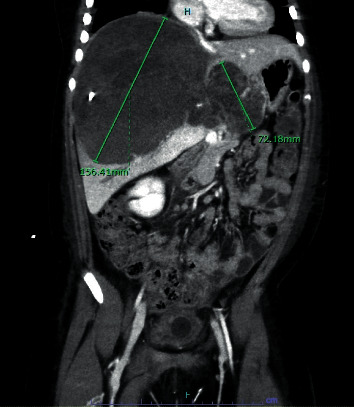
Coronal contrast-enhanced abdominal CT demonstrating large hepatic tumor with in situ drain in the right hepatic lobe.
